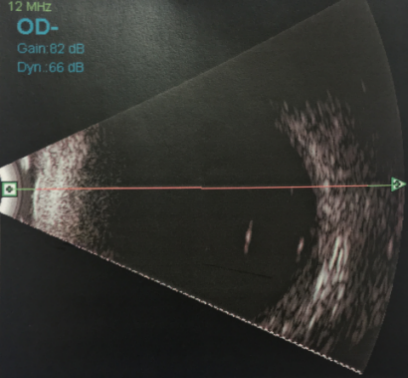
B-scan ultrasound of the right eye with vitreous opacity
